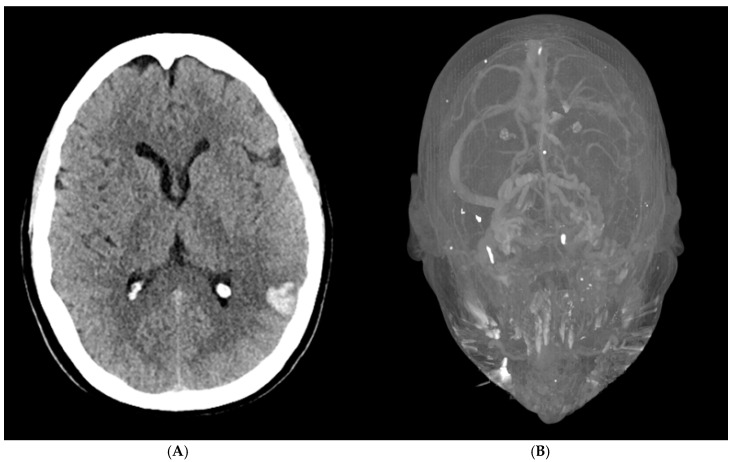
(A) Non-contrast CT (bottom) reveals intraparenchymal hemorrhage in the left temporoparietal region, consistent with hemorrhagic transformation due to CVST. (B) CT venography (top) shows cerebral venous sinus thrombosis (CVST) with thrombosis of the dural sinuses.
